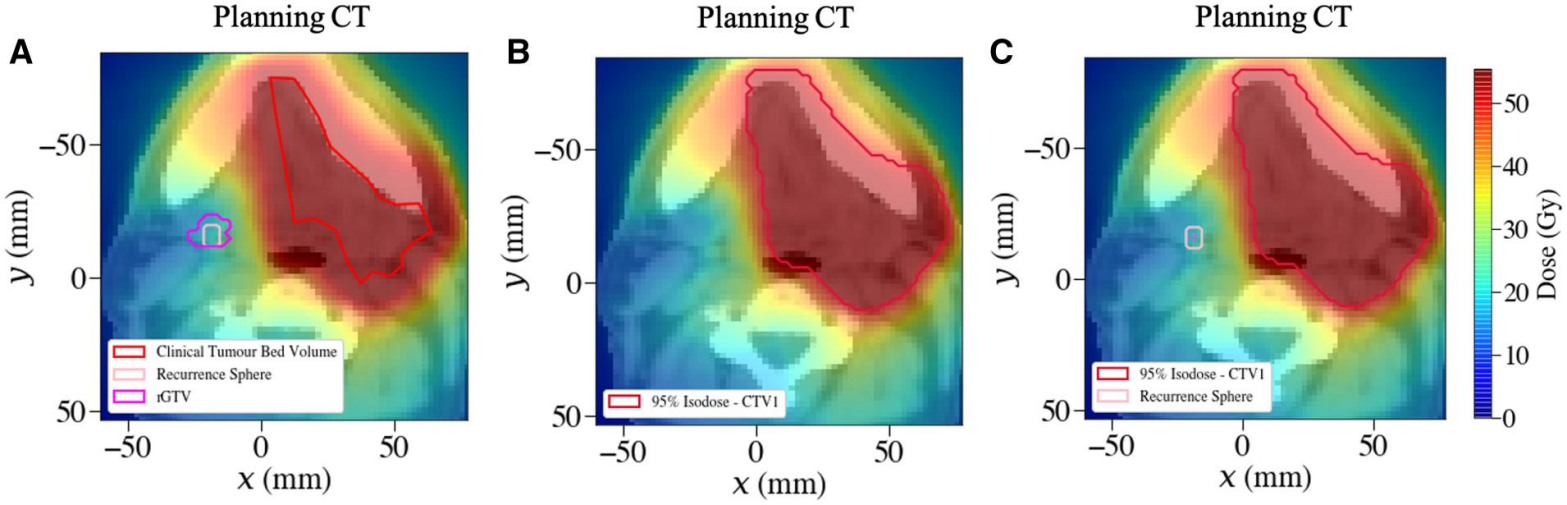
Structure sets for Patient Y. (A) Clinical tumour bed volume, mapped rGTV volume as outlined by the radiation oncologist, and the relapse sphere generated around the mapped rGTV centroid. (B) 95% dose structures generated from the planned treatment dose distribution for 95% dose to high-risk region (CTV1) to capture dose spread outside the clinical CTV. (C) The planning CT with overlaid 95% dose structure and spatially mapped recurrence sphere and centroid.
